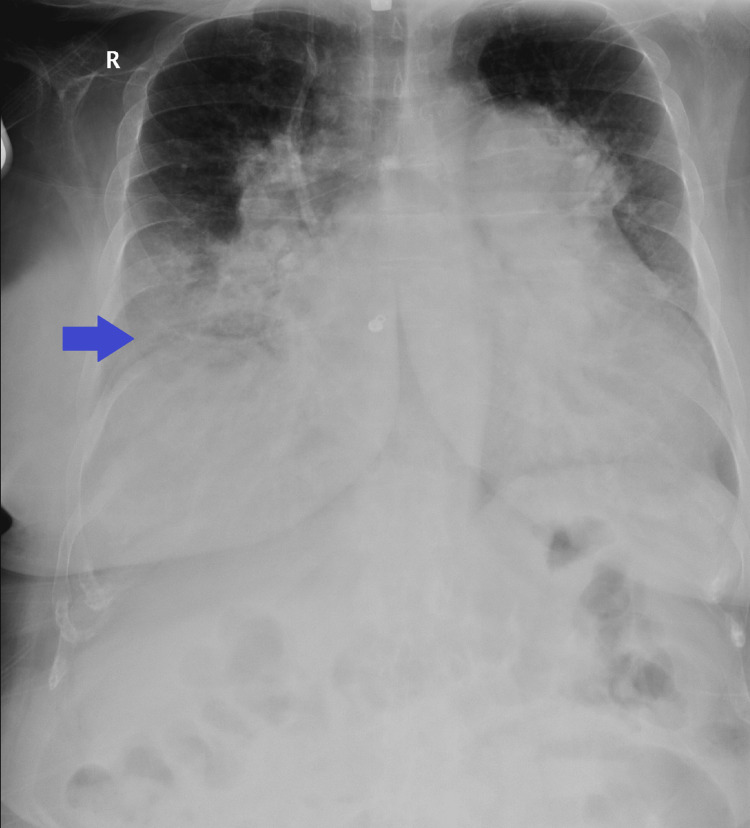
Chest X-ray showing cardiomegaly and right lower lobe infiltrates (blue arrow).
